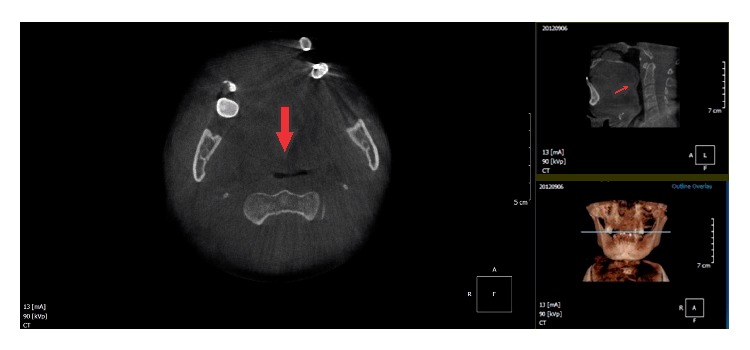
Cone-Beam Computed Tomography. Axial section showing extensive lesion on the buccal floor (in the sublingual space), demonstrating hypodense areas and almost airway obstruction (red arrows).
